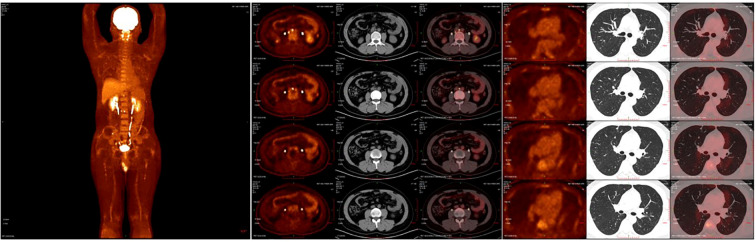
(A) The PEC-CT scan reveals the systemic tumor burden after 4 cycles of chemotherapy. (B) The PET-CT scanning revealed that glucose metabolism was elevated in the vicinity of the right external iliac vessel and the abdominal para-aortic lymph nodes. (C) The PET-CT scan showed no lesion of increased glucose metabolism in both lungs. PET-CT, Positron Emission Tomography - Computed Tomography.
